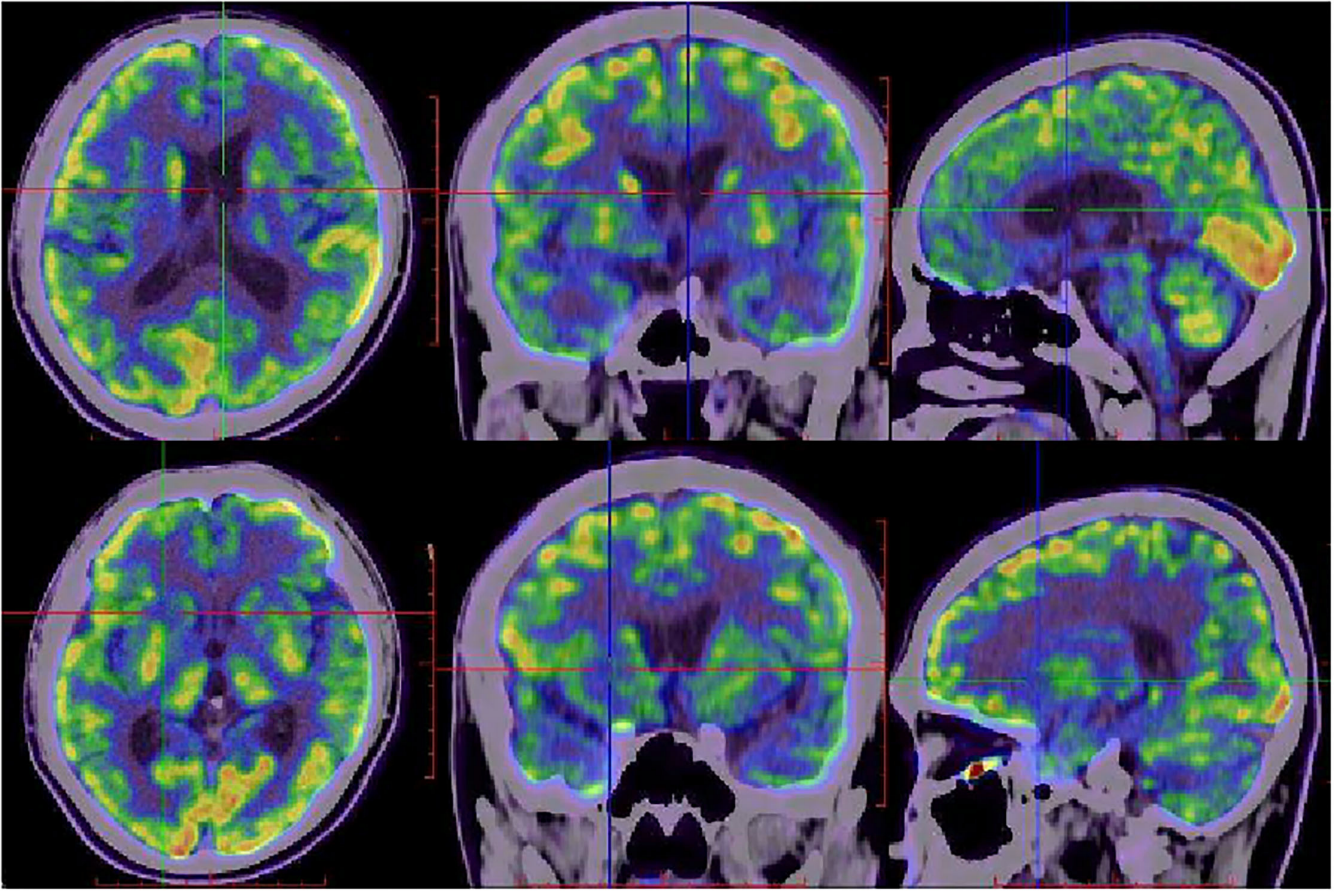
FDG-PET images of the proband at 20 months after onset. FDG-PET showed hypometabolism in bilateral frontal, parietal, temporal lobes, bilateral caudate nucleus, putamen, and thalamus, with right lateralized basal ganglia hypometabolism.
